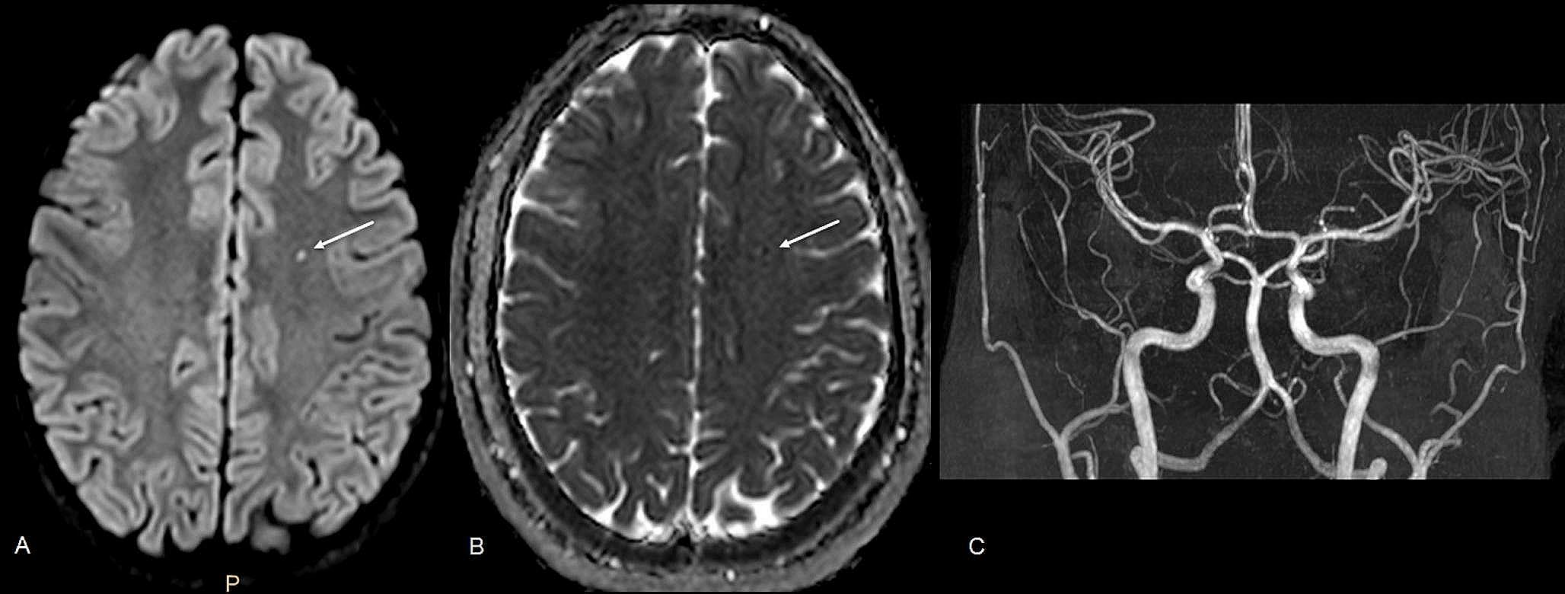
1.5 Tesla brain MRI with diffusion-weighted sequence displaying a punctiform lesion at b1000 (A), exhibiting restricted diffusion on ADC map (B), and no evidence of brain vessel occlusion on 3D Time Of Flight (TOF) imaging (C) Diagnosis: recent small ischemic stroke affecting the left frontal lobe
